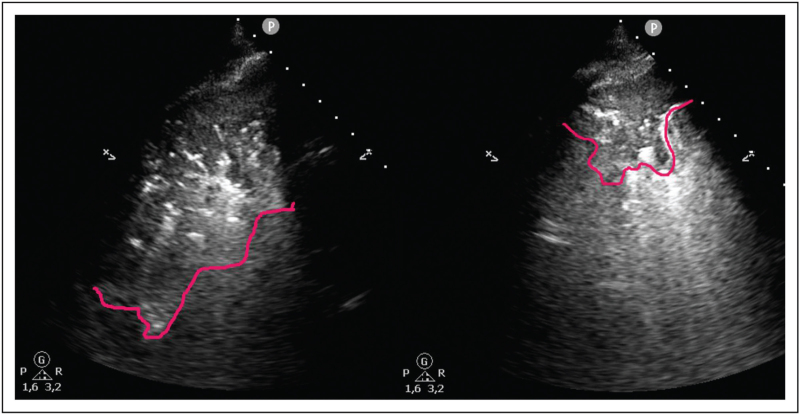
Effect of PEEP on reaeration of the lung. Increase of PEEP can result in recruitment of lung tissue which can be seen on lung ultrasound as a smaller consolidation when PEEP is increased from PEEP 5 (left) to PEEP 15 (right). The lines indicate the border of the consolidations. PEEP, positive end-expiratory pressure.
